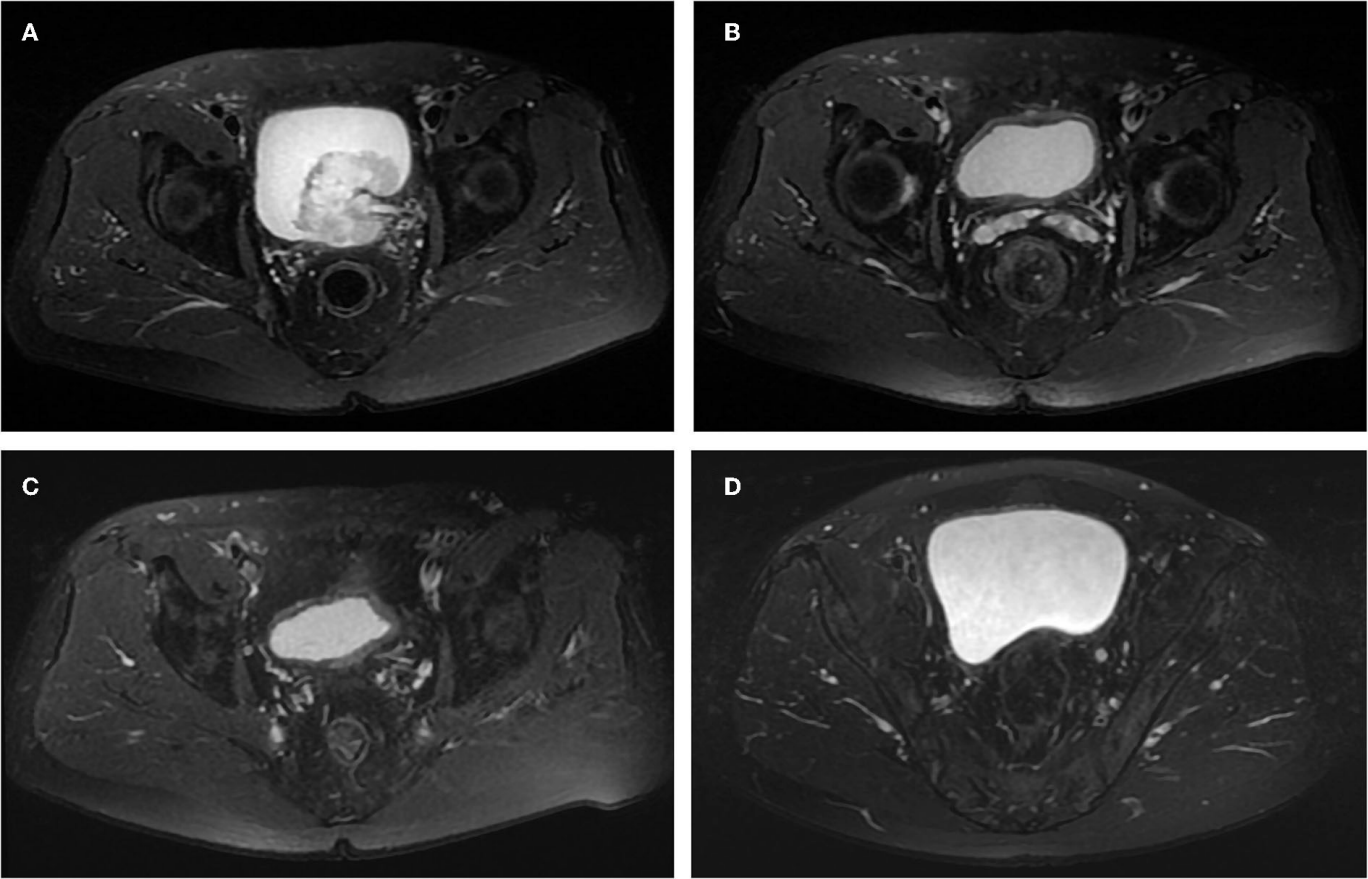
Images of the case 2 patient throughout the treatment. (A) Before treatment, pelvic MRI cross-sectional T_2_W showed a lump in the left posterior wall of the bladder and mild dilatation of the left ureter. (B) After the first maximal TURBT and four cycles of adjuvant chemotherapy plus tislelizumab, no tumor was seen on pelvic MRI cross-sectional T_2_W. (C) After the second maximal TURBT and gemcitabine intravesical therapy, no tumor was seen on pelvic MRI cross-sectional T_2_W. (D) On April 12, 2022, the last follow-up, no tumor was seen on pelvic MRI cross-sectional T_2_W.
